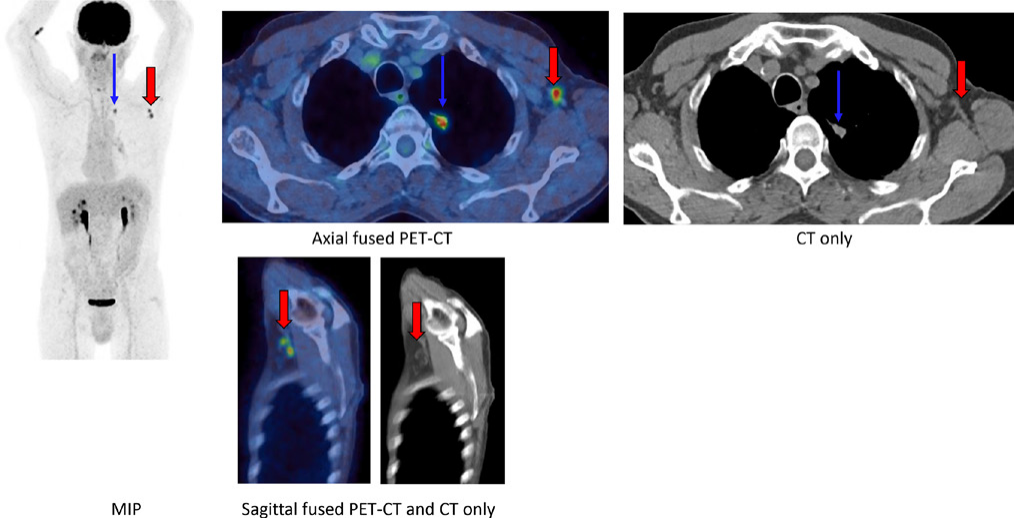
FDG PET-CT (MIP, axial fused PET-CT, axial CT only, sagittal fused PET-CT and sagittal CT only) demonstrated intense asymmetric uptake in two normal-sized left axillary lymph nodes, containing normal fatty hilum (red arrows). No uptake was seen in deltoid musculature. Left upper lobe lung nodule is also demonstrated (blue arrows).
